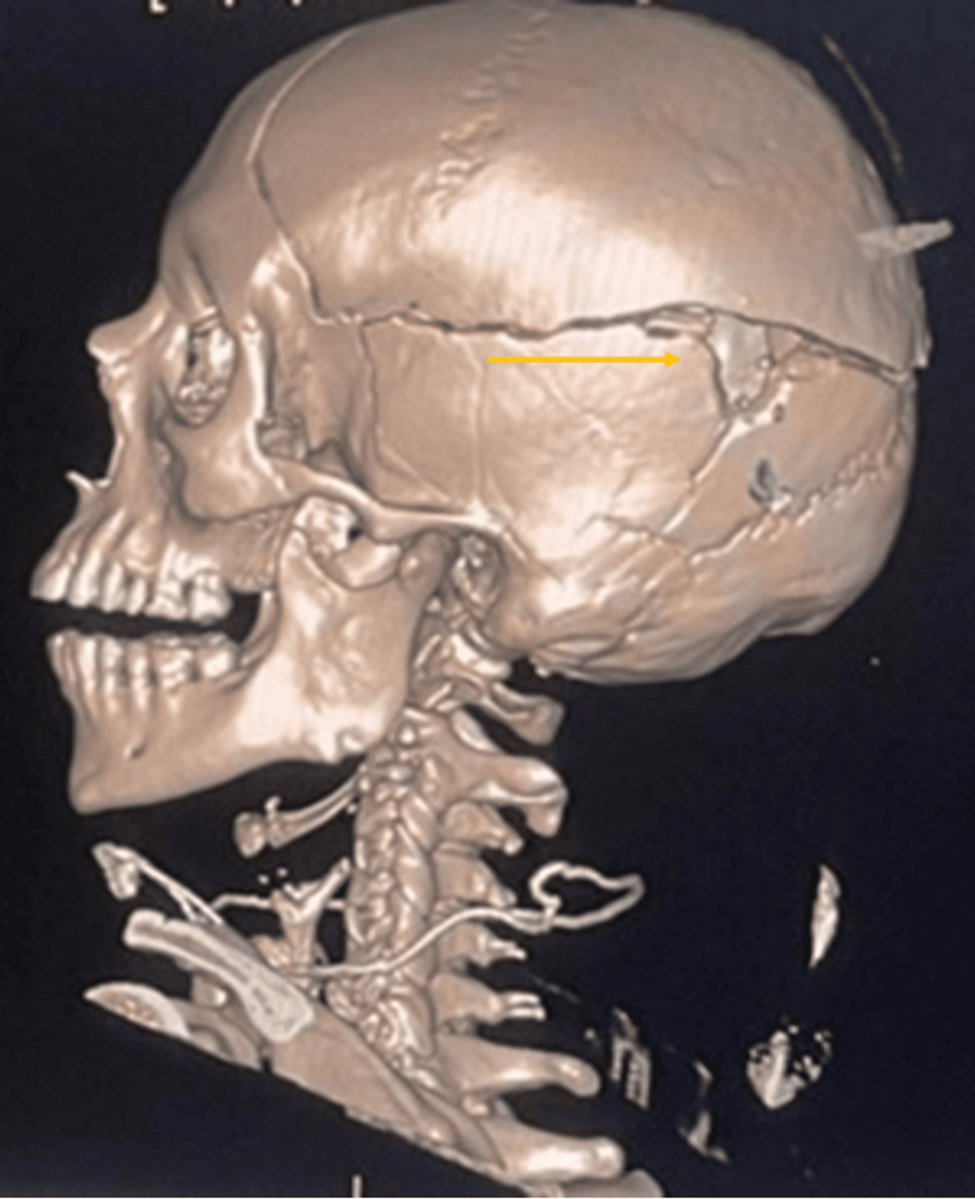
CT image of the cranial trauma showing a gunshot exit wound in the left parieto-temporal region. The image highlights adjacent bone fractures consistent with a high-energy firearm injury
